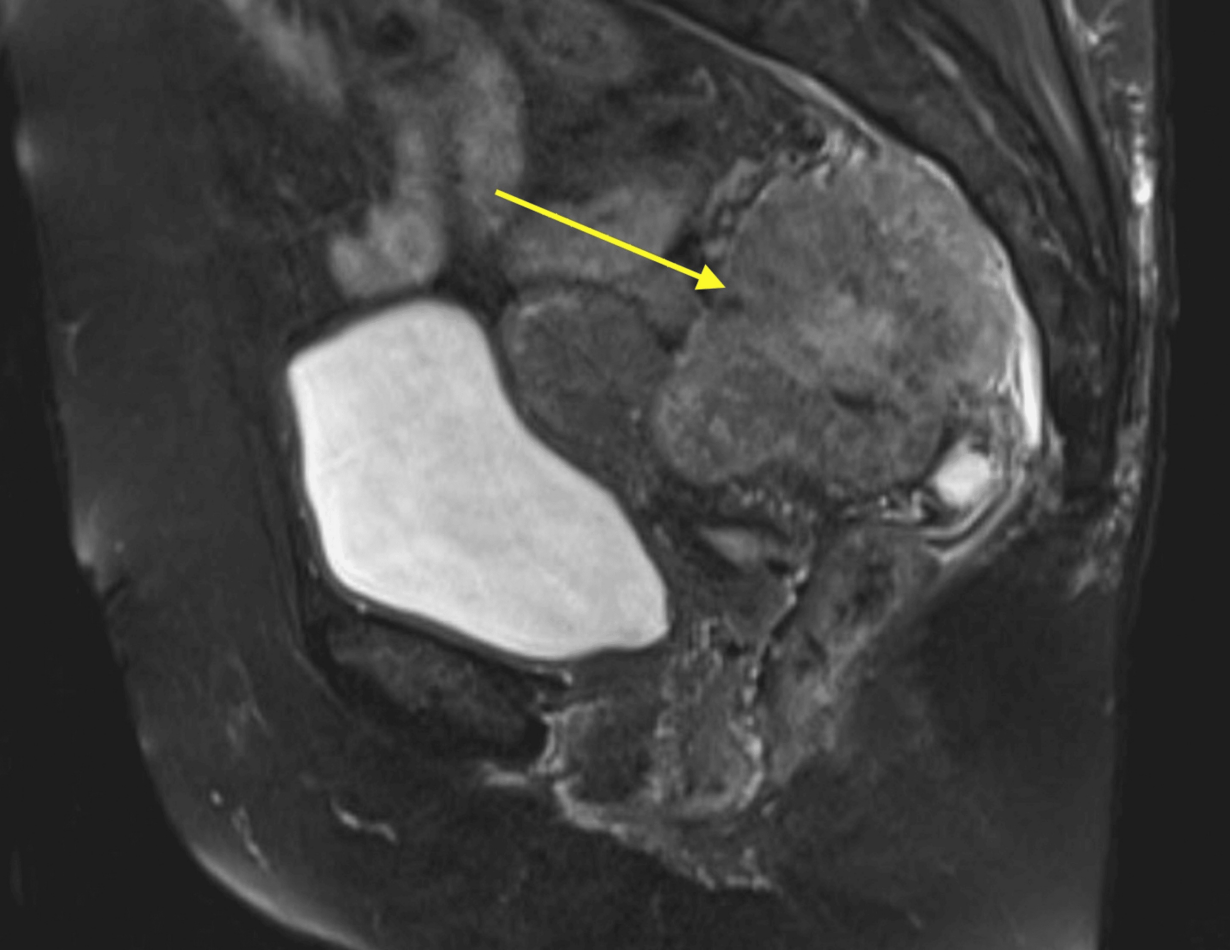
Sagittal MRI demonstrating dominant T2 pelvic mass (yellow arrow) with effect on surrounding structures. MRI: magnetic resonance imaging
